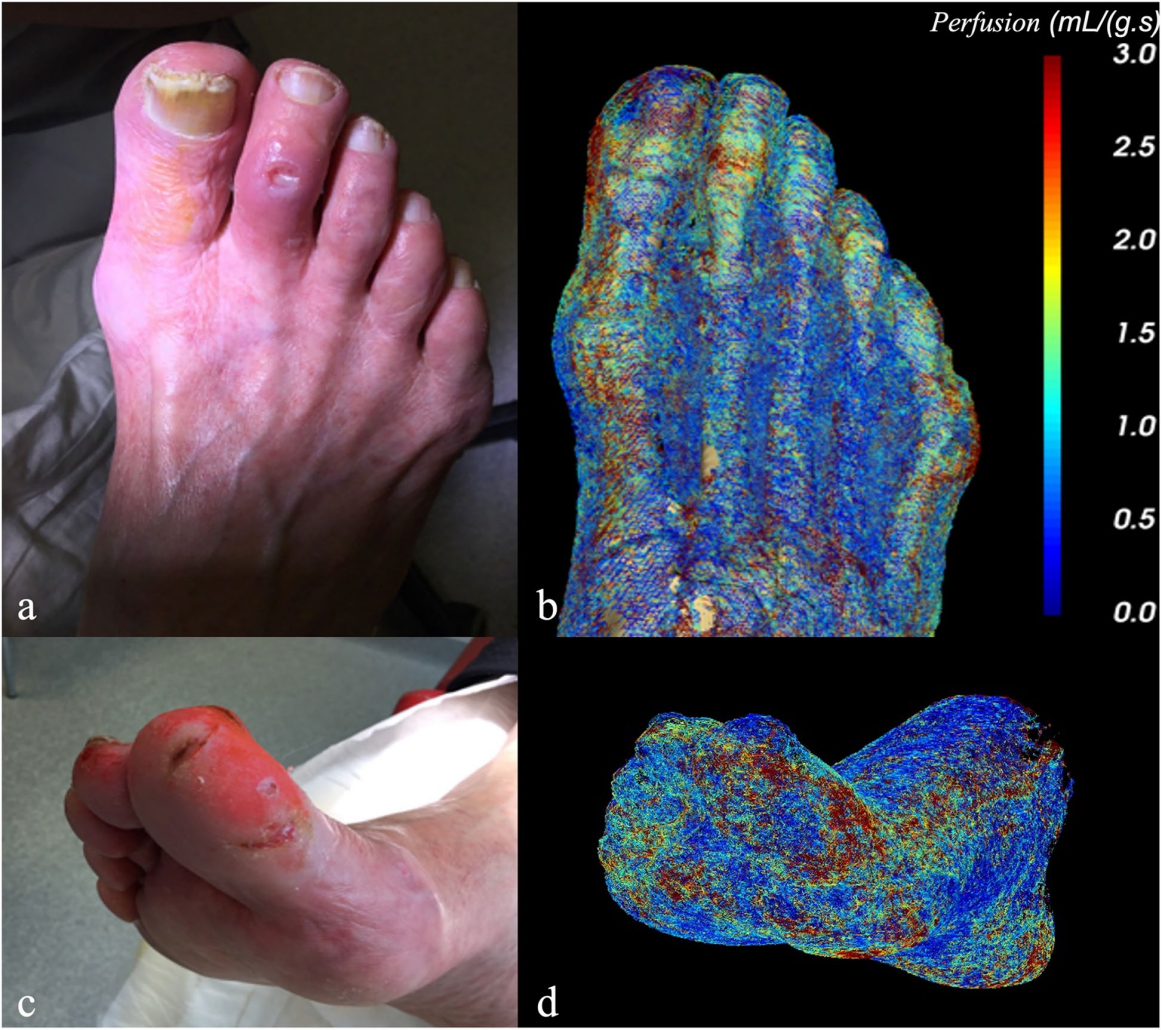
a, c Participant number 3 presenting several necrotic ulcers at the first and second toe. b, d Volume rendering of the parametric blood flow map indicating the perfusion values (mL/g/s) derived from 4DCT by means of color-coding: low perfusion values are depicted in blue, high values in red. *4DCT* Four-dimensional computed tomography
